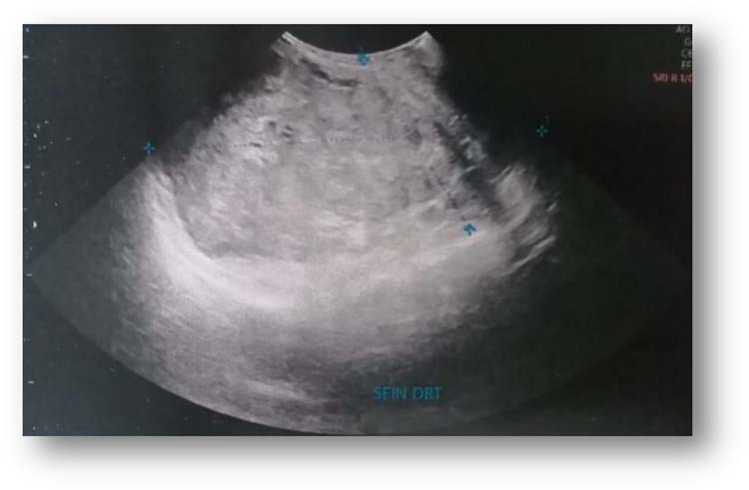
Ultrasonographic examination: large heterogenous hypoechoic mass with polylobed contours of 18 × 16 × 10 cm.
